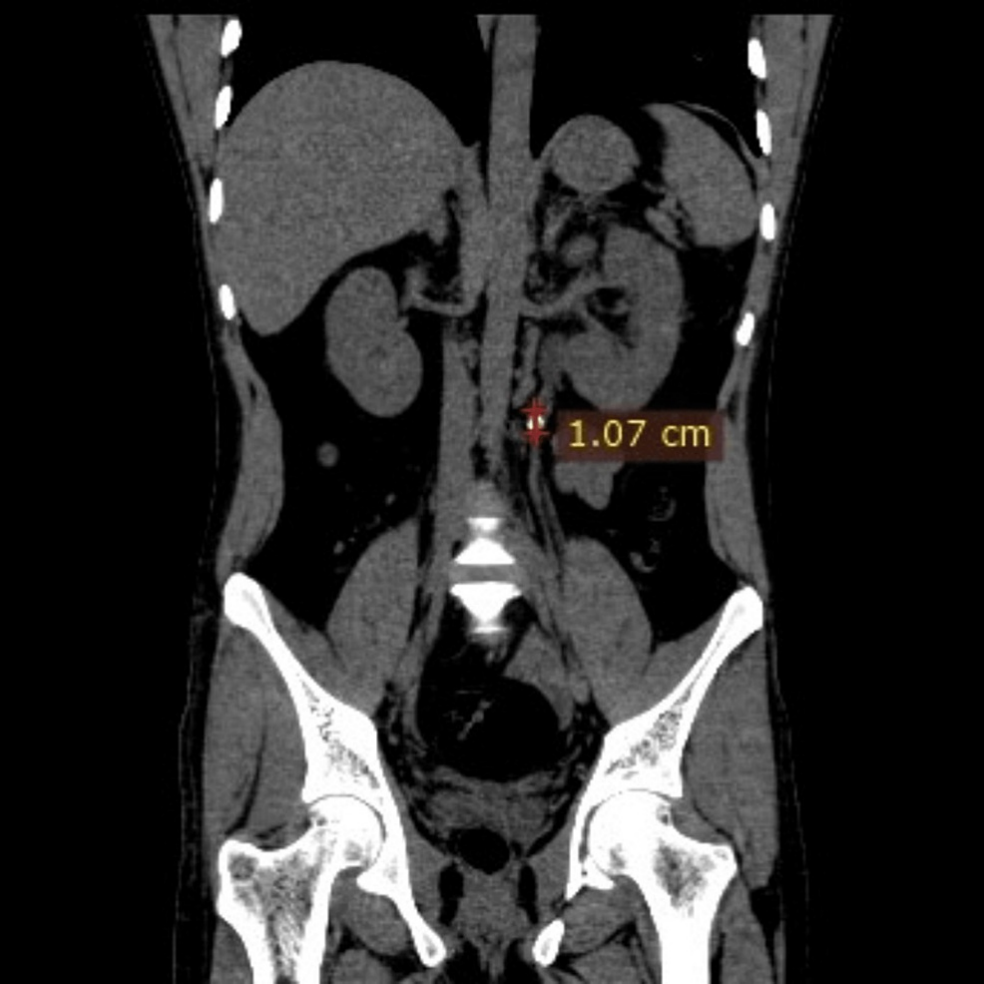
Coronal CT section of the abdomen shows a left ureteric calculus and its dimensions.
